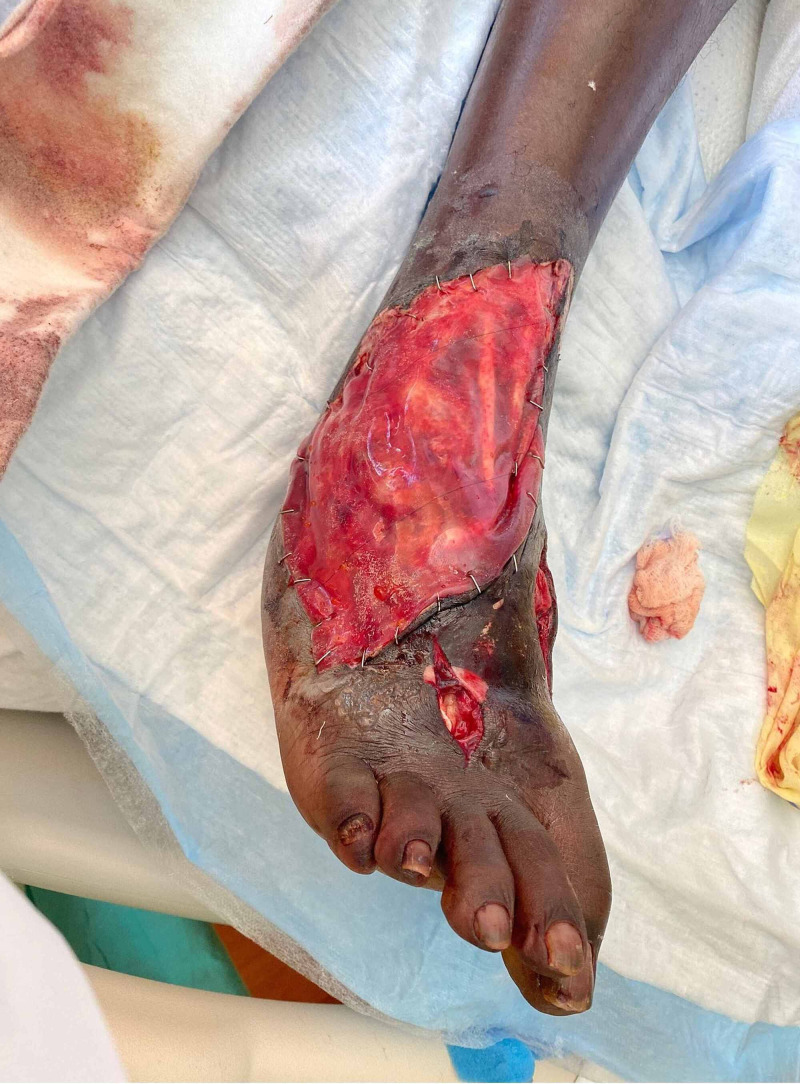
Patient's right lower extremity after revascularization and compartment release Shown here is the patient's right lower extremity after revascularization of the dorsalis pedis with a saphenous vein interposition graft and coverage with Integra^TM ^(Integra LifeSciences, Princeton, NJ). Compartment release was performed in the interdigit space. At this time the tissue was dusky but with Doppler signals in the first interdigit webspace.
